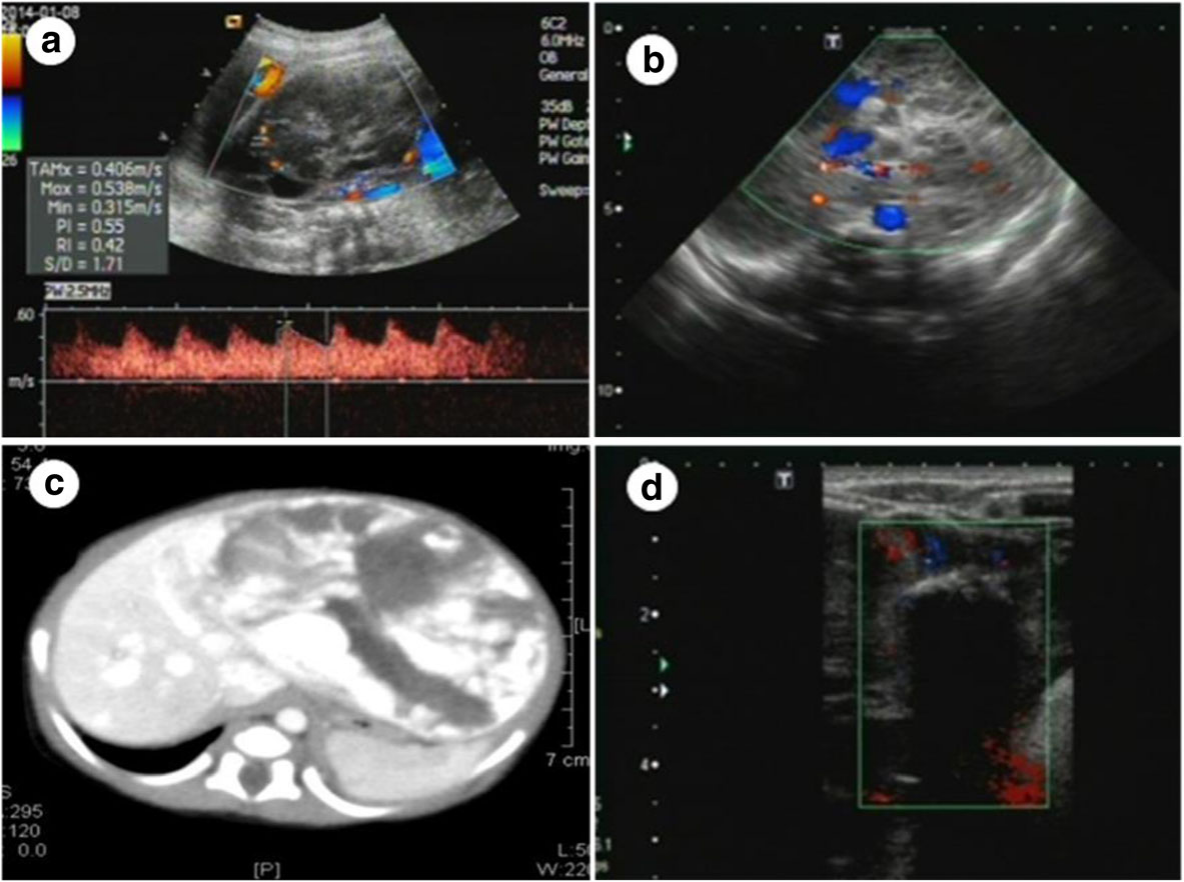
Fetus with multiple hemangiomas in the whole liver (fetus 2). a Largest hypoechoic lesion, measuring 92 × 56 × 80 mm and located in the left liver lobe. Color Doppler ultrasound shows a ring of blood flow at the edge of the lesion and stars intralesion. Pulse Doppler ultrasound suggests that the resistance index is 0.42. b Ultrasound on the day of birth shows that the large lesion has a clear border and an internal grid-shaped region. The portal vein was unobstructed, and its inner diameter was normal. c Postnatal computed tomography (CT) shows the large lesion in the left lobe and several smaller lesions in the right lobe. The density of the mass was low but not uniform. On contrast-enhanced CT, the edge of the lesion increased in intensity in a lattice-like pattern in the early phase of the scan, and the center of the mass gradually increased in intensity during the delay phase; there was no enhancement in the low-density zone. d Two years after treatment of the neonate with propranolol and dexamethasone, the lesion was 19 × 19 × 10 mm in size. Calcification plaques were observed in the lesion, and the intrahepatic and the extrahepatic bile ducts were not dilated
